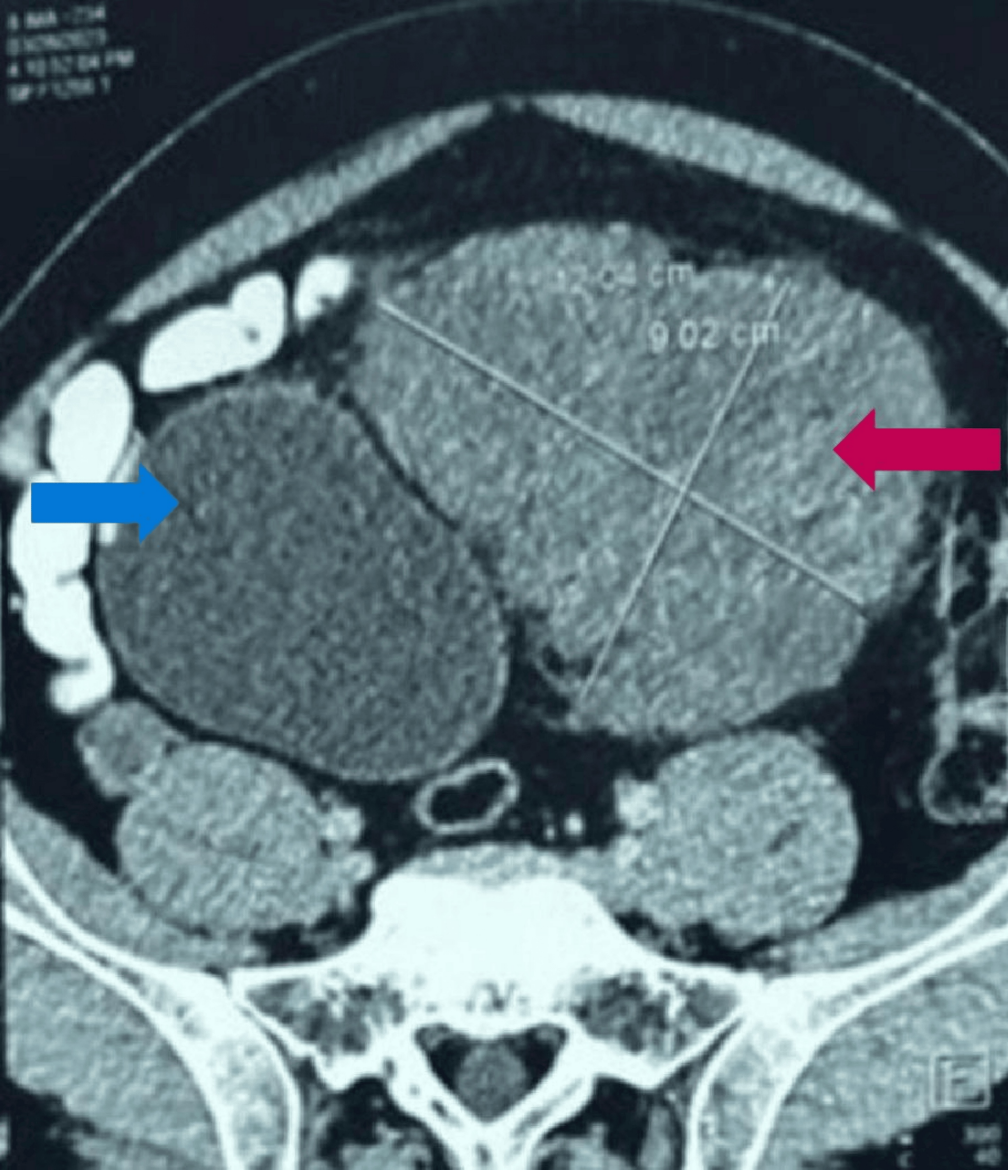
Transverse section of the abdominal CT scan, showing the tumor (red arrow) arising from posterior to the anterior abdominal wall without any infiltration to the urinary bladder (blue arrow) CT: computed tomography
